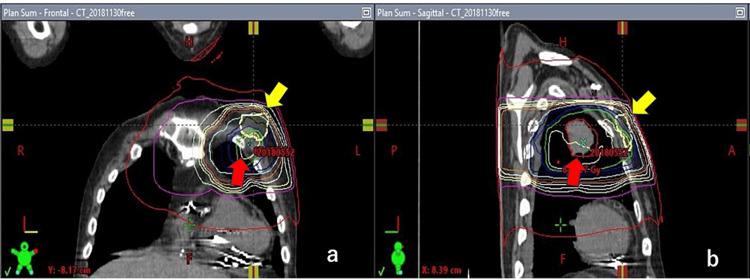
CT before radiation therapy. Exposure dose of the radiation to the pacemaker (yellow arrows) is shown. Red arrows indicate the tumor of the left lung (a: frontal view; b: lateral view).
